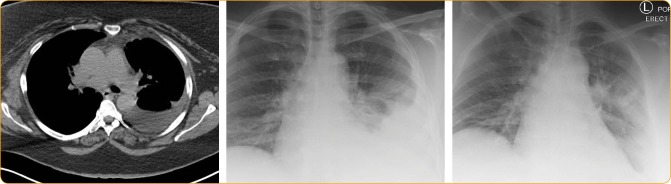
CT scan of the chest (left) shows pleural effusion. Chest x-rays done on the same day as the CT scan, predrainage (middle) and postdrainage (right).
